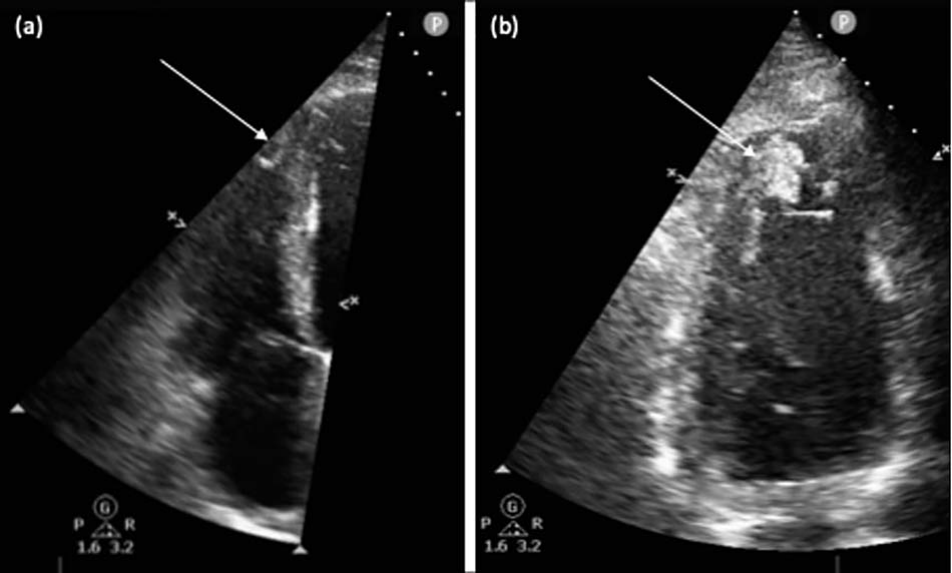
Transthoracic echocardiogram. (a) Focused RV view with thrombus visualized in the apex (arrow). (b) Two-chamber view of the LV with thrombus visualized in the apex (arrow).
